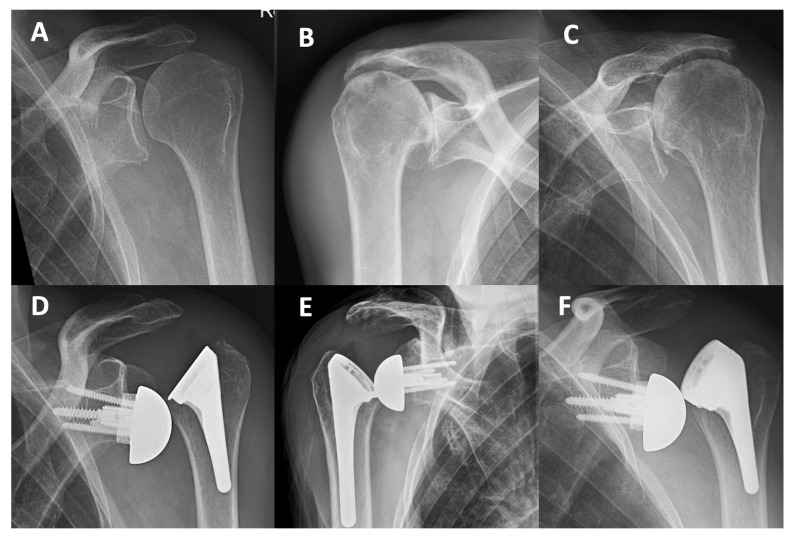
Preoperative radiographs of three patients who sustained dislocations (A–C). Superior–lateral dislocations of short Perform stems in combination with standard non-retentive liners (D–F).
